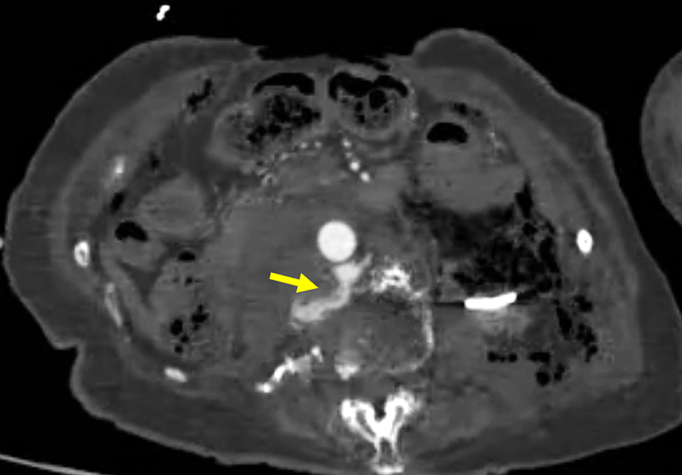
Contrast-enhanced computed tomography showing extravasation from a pseudoaneurysm to the second lumbar vertebra (arrow).
